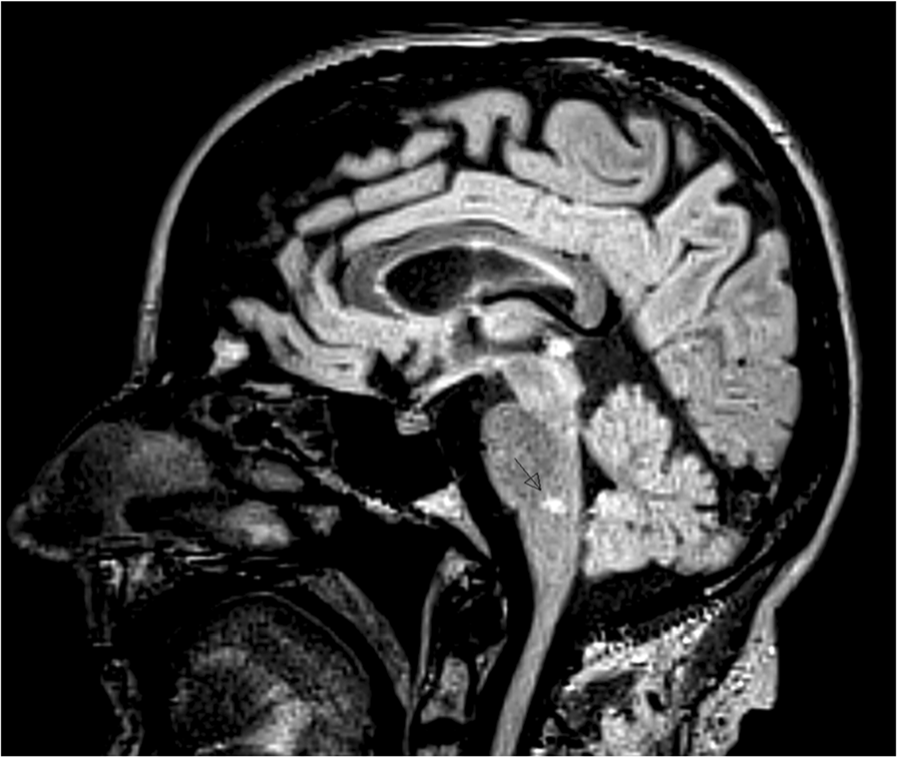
Sagittal 3D FLAIR MR image acquired at 1.5 Tesla (Siemens Avanto) showing a hyperintense lesion in the medulla oblongata (arrow). FLAIR = fluid attenuated inversion recovery.
